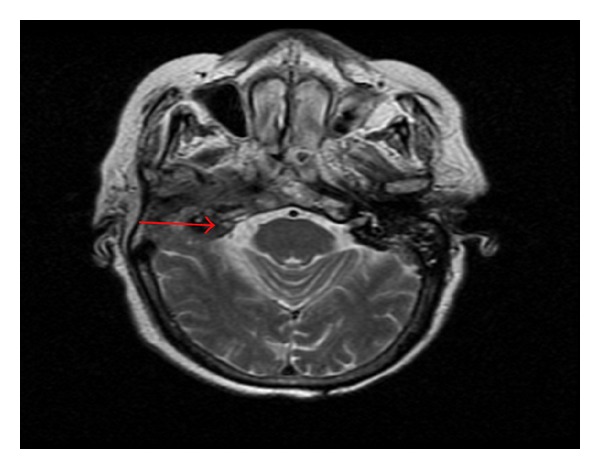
Neck soft tissue Magnetic resonance imaging showing heterogeneous signal abnormality and enhancement centered about the right external auditory canal and middle ear region. The arrow depicts spread of the tumor into the middle ear.
